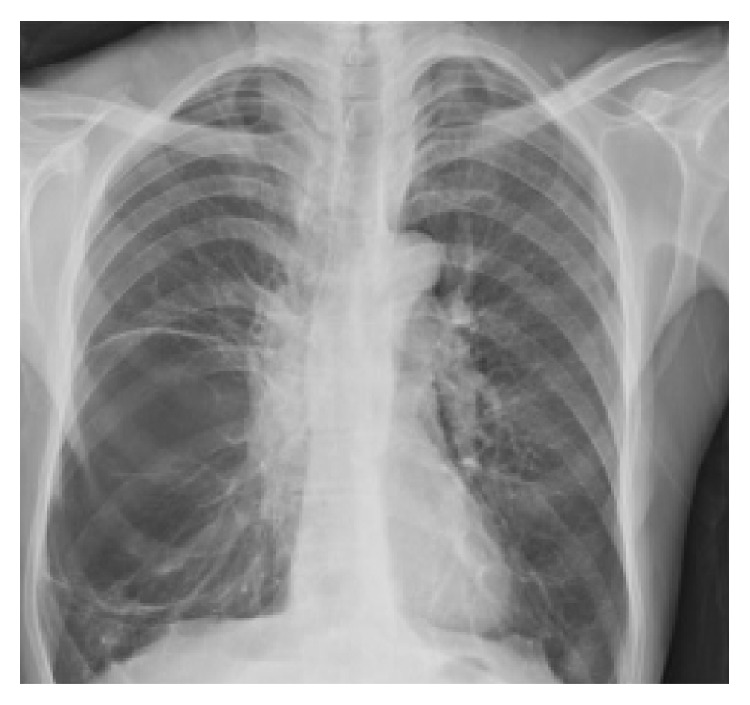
Pretreatment chest X-ray.
